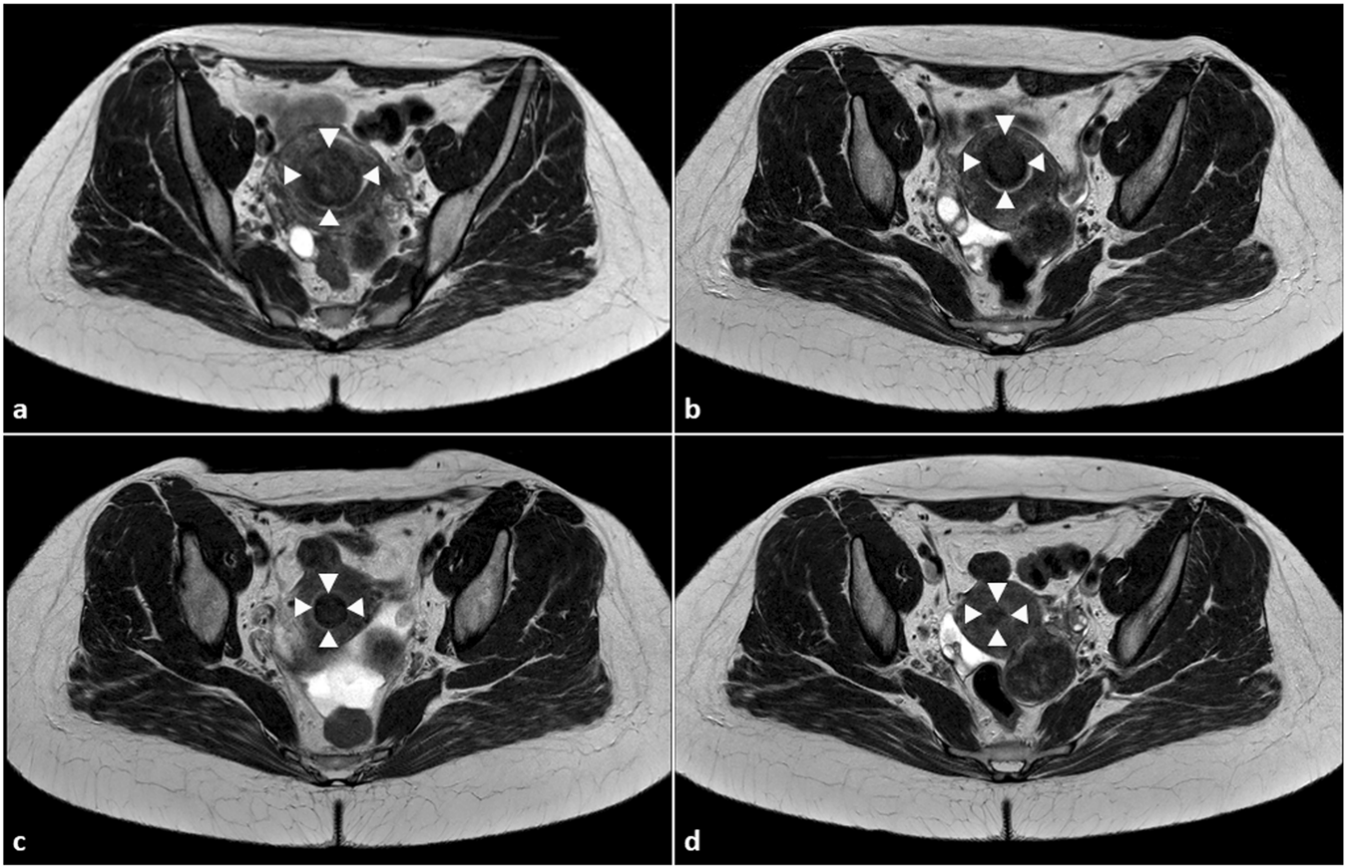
MRI images (transverse T2-weighted sequences) of the pelvis of a 41-year-old premenopausal patient are shown. The patient presented with severe fibroid-associated symptoms (hypermenorrhea, dysmenorrhea, anemia, pelvic pain and feeling of pressure). The predominant target fibroid was located in the fundus of the uterus. In the 1-month follow-up, a significant reduction in symptoms and fibroid volume was observed. a Large predominant target fibroid in the fundus of the uterus (white arrows) prior to HIFU treatment. b Significant fibroid shrinkage was observed at 3 months with a 54% reduction in lesion volume. c, d Fibroid volume reduction of approximately 85% at 6 months (c) and 94% at 1 year (d) post-HIFU
